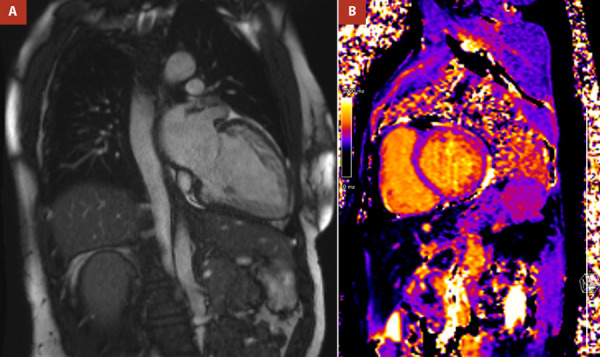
Cardiac MRI. Cine images in two chambers showing hypertrabeculation (A) and native T1 mapping (B) showing normal values.
